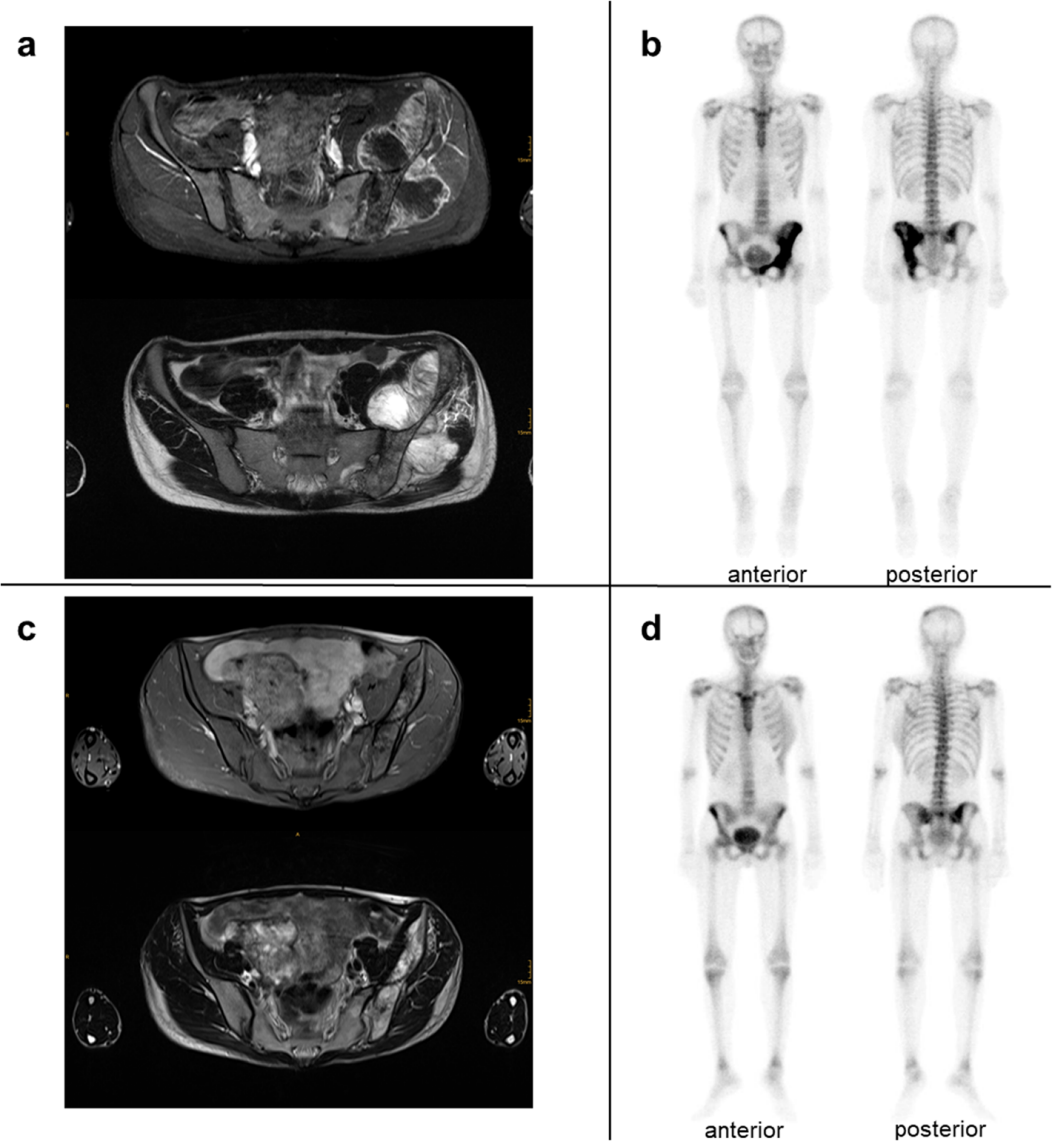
Imaging results of patient at diagnosis (a/b) and last follow-up 26 months later (c/d). a Axial MRIs of the pelvis showing chrondroblastic osteosarcoma of the left Os ileum with infiltration of gluteal muscles, left iliac and obturatorius internus muscle, periosteous soft tissue, Os sacrum and Os pubis with strong contrast enrichment. T_1_-weighted transversal MRI with i.v.-contrast-medium (gadoteriol, Prohance®, Bracco) and fat saturation (above) and T_2_-weighted transversal MRI (bottom). b Aside from intensive bone turn over at the left hemipelvis, no other suspicious bone lesions are observed in initial bone scan. c Axial MRIs of the pelvis showing residual proportions of chrondroblastic osteosarcoma of the left Os ileum with decreasing contrast enrichment and signs of sclerosis and ossification of small soft tissue component. T_1_-weighted MRI with contrast agent and fat saturation (above) and T_2_-weighted MRI (bottom). d Bone scan demonstrating a normalisation of bone turn over at the left hemipelvis. MRI could rule out an initially suspected left parietal metastasis. Aside from an accentuation of the right sacroiliac joint due to incorrect weight bearing, no other suspicious bone lesions were observed
